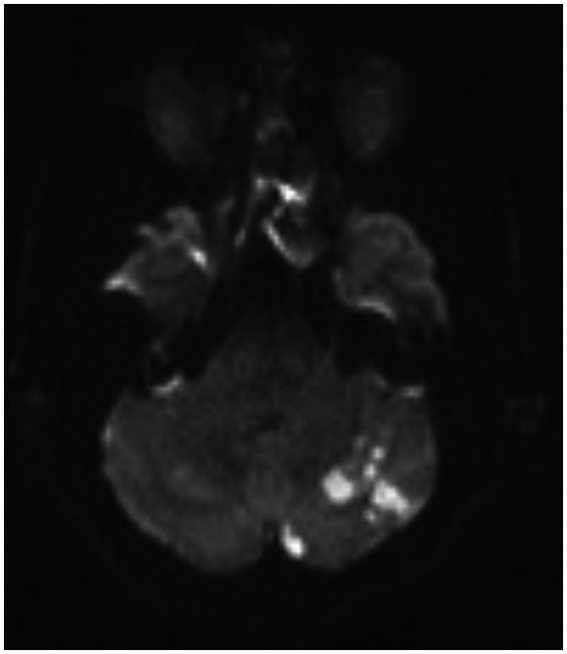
A repeat MRI of the brain showing increased burden of diffusion restriction in bilateral cerebellar hemispheres.
